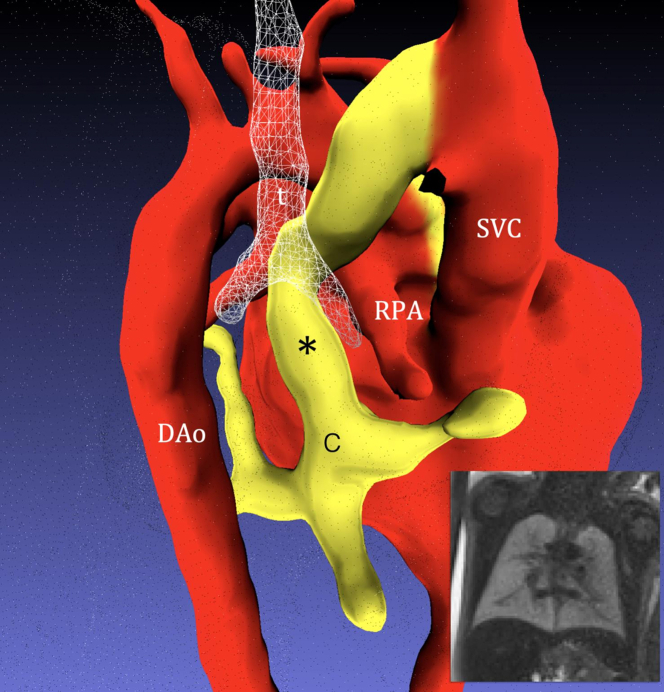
Segmentation From Motion-Corrected Slice-to-Volume Magnetic Resonance Imaging Data (Posterior Rightward Projection) at 34 Weeks The confluence (C) drains via an ascending vein (asterisk) that passes between the right main bronchus (white mesh) and right pulmonary artery (RPA) before draining the innominate vein/superior vena cava (SVC). Inset shows single-shot fast spin echo image of the fetal lungs, demonstrating heterogeneity in the lung parenchyma with small cystic areas suspicious for pulmonary lymphangiectasia. DAo = descending aorta; t = trachea.
